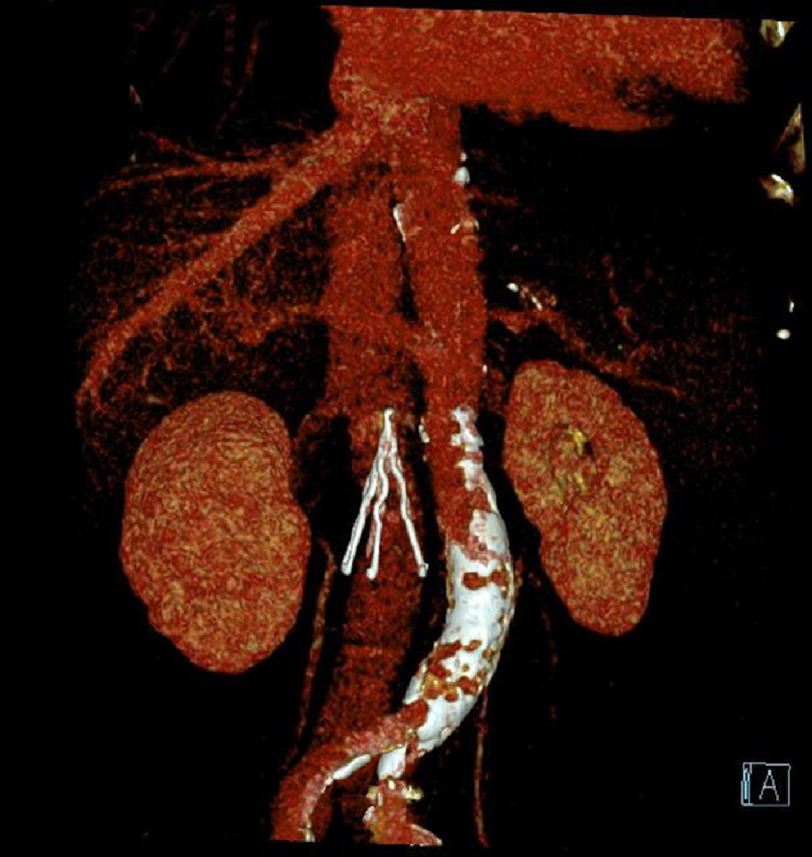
CT shows inferior vena cava filter.
